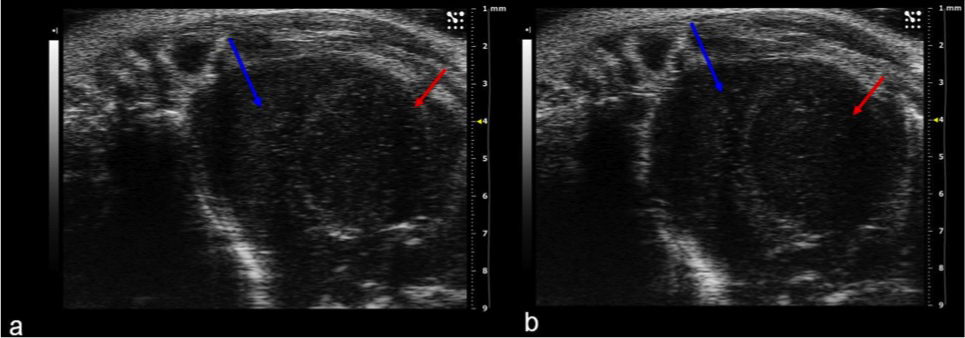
(a, b) Apical two-chamber view showing right ventricle (red arrow) and left ventricle (blue arrow) during systole (a) and diastole (b). The ejection fraction and shortening fraction were calculated with this view.
